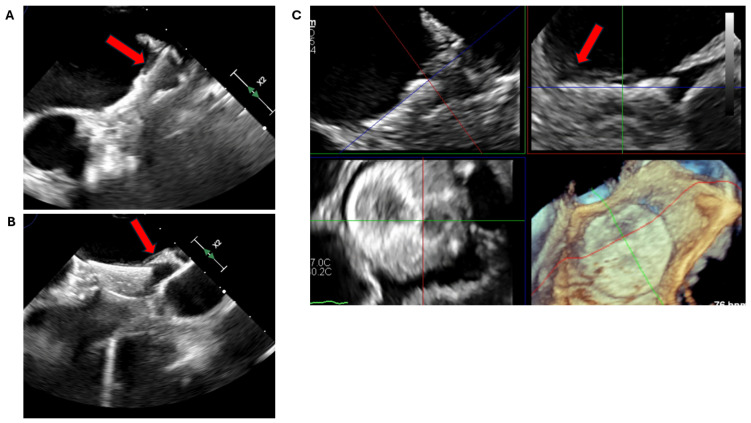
The same patient in Figure 18 was assessed with 2D (A,B) and 3D TOE (C). This shows a well-organised, thin laminar layer of echo-bright lesion partially covering the disc on the atrial aspect of the device (red arrows), in continuity with the LA endothelium and in keeping with normal endothelialisation. TOE: Transoesophageal echocardiogram; 2D: Two-dimensional; 3D: Three-dimensional; LA: Left atrium.
